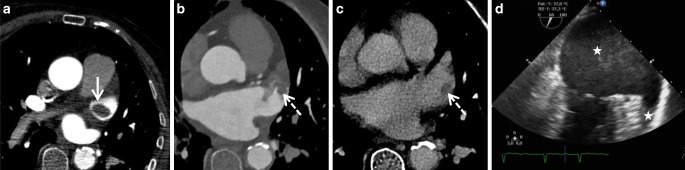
a–c Computed tomography in short-axis view of the heart and d transesophageal ecocardiography in four-chamber view. In this patient a thrombus decreased over time even without administering lysis therapy due to early cerebral infarct bleeding. In the initial examination, an oval contrast agent recess with a diameter of 24 mm in the sense of a thrombus can be seen (a, *white arrow*). At cardiac CT examination (b, c) 17 h after initial imaging, in the arterial (b) and venous phase (c) a smaller thrombus (10 mm, *white dotted arrows*) could be detected. Note the LAA circulatory disorder with triangular contrast agent sparing in the arterial phase (b). The TEE on day 7 shows spontaneous echo contrast (SEC) or smoke-like echo (*white stars*) without solid thrombus detection (d)
